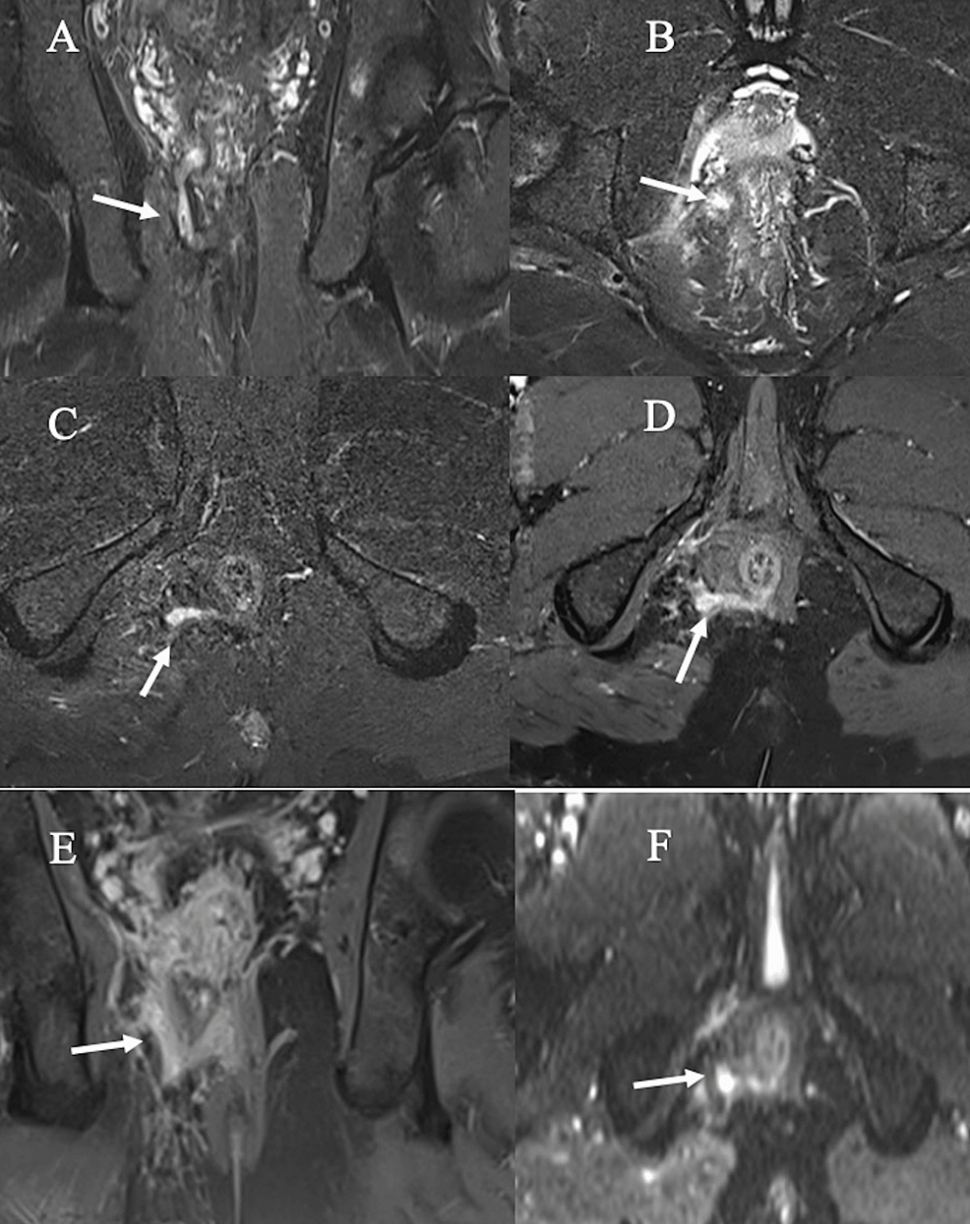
Grade 5 supralevator fistula: (A-B) Coronal STIR and axial post-contrast fat-suppressed T1-WI showing Grade 5 supralevator fistula. (C-F) Axial STIR, axial, coronal post-contrast fat-suppressed T1-WI, and DWI, respectively, showing trans-sphincteric medial ramification, which is crossing external sphincter with restricted diffusion on DWI. DWI: diffusion-weighted imaging, STIR: short tau inversion recovery
